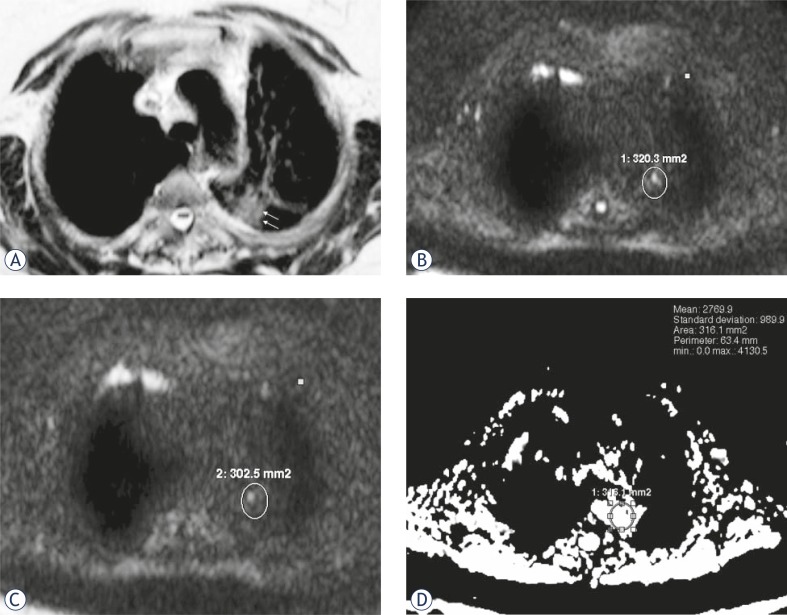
A 32-year-old woman with bacterial pneumonia. (A) Axial T2-weighted MR image shows ill-defined peripheral mass in the superior segment of the lower lobe of the left lung (arrows). (B) diffusion-weighted magnetic resonance image with b value of 500 sec/mm^2^ shows minimal hyperintense mass (SI = 203). (C) diffusion-weighted magnetic resonance image with b value of 1000 sec/mm^2^ shows hyperintensity of the mass is less remarkable (SI = 162). (D) ADC map shows ADC value is (2.76×10^−3^) sec/mm^2^.
